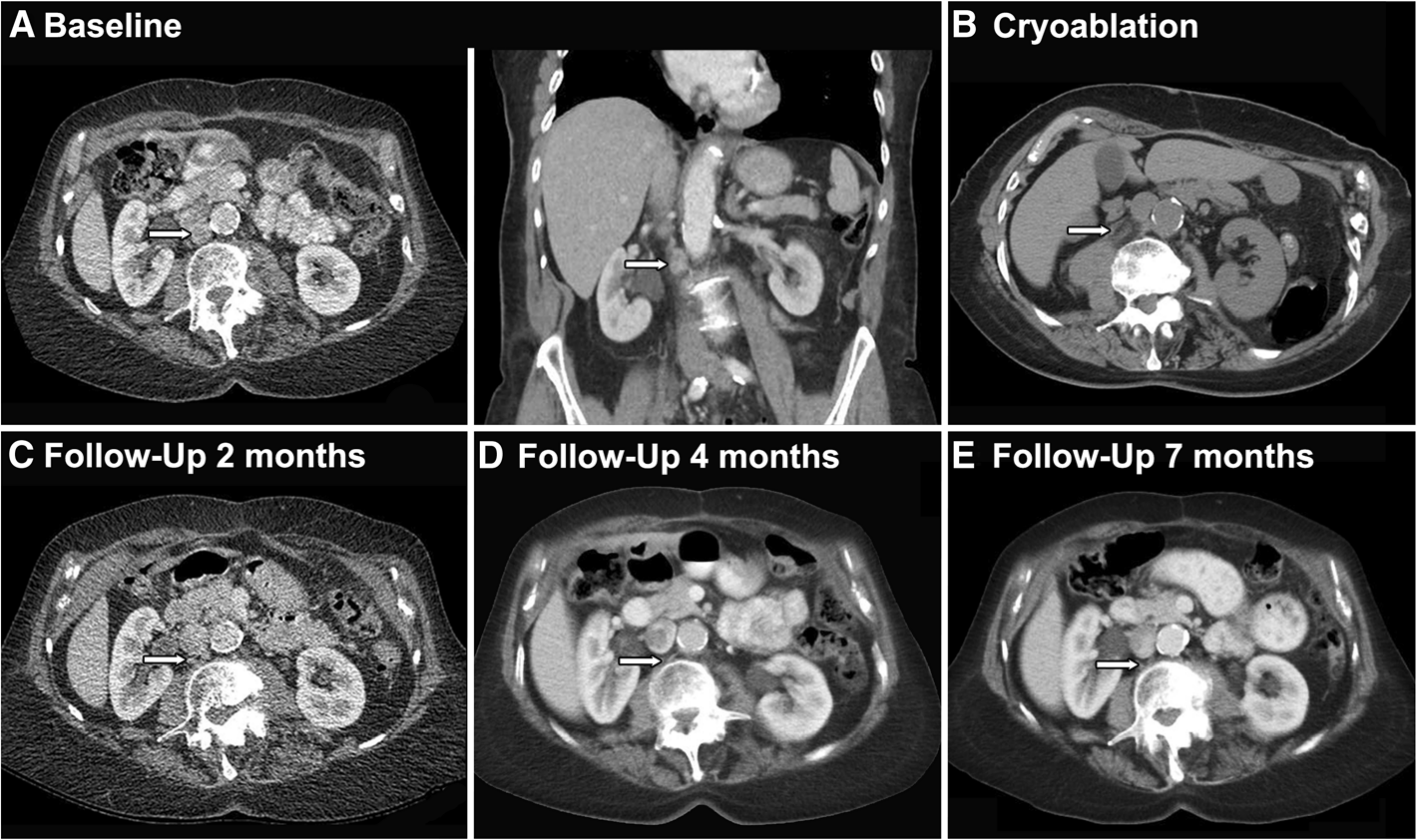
Imaging of patient undergoing combination anti-PD-L1 and anti-CTLA-4 immunotherapy. a Baseline CT of the abdomen demonstrated an aortocaval lymph node. b Axial CT obtained during cryoablation demonstrates an ice ball. c - e Follow-up CTs showing completely responsive lymph node after partial cryotherapy
